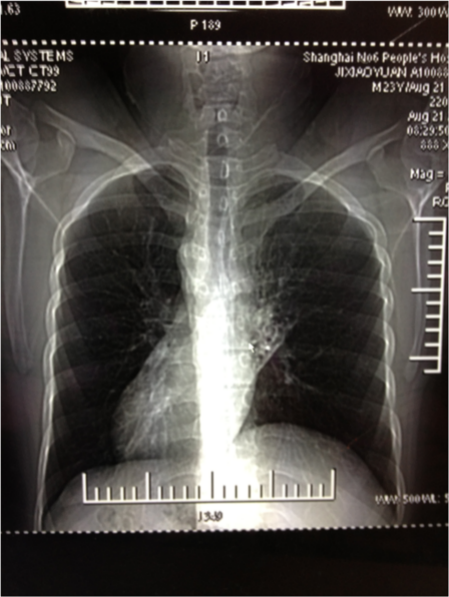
Chest computed tomography scan showing dextrocardia
